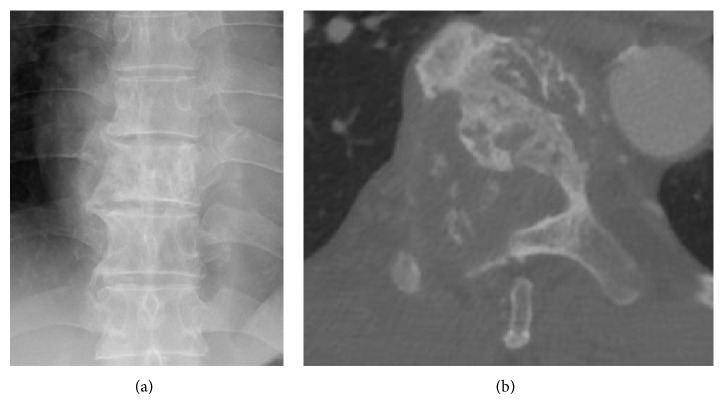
Preoperative images showing a vertebral tumor destroying the T9 vertebra and right pedicle. (a) Preoperative anteroposterior radiograph, (b) CT of the T9 vertebra.
